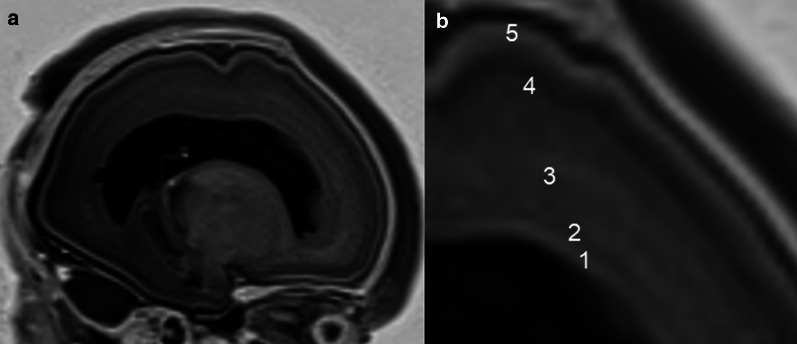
23-week foetus with normal cerebral lamination. a, b Sagittal T1-weighted images show five laminar compartments: (1) ventricular zone; (2) periventricular zone; (3) intermediate zone; (4) subplate zone; (5) cortical plate
